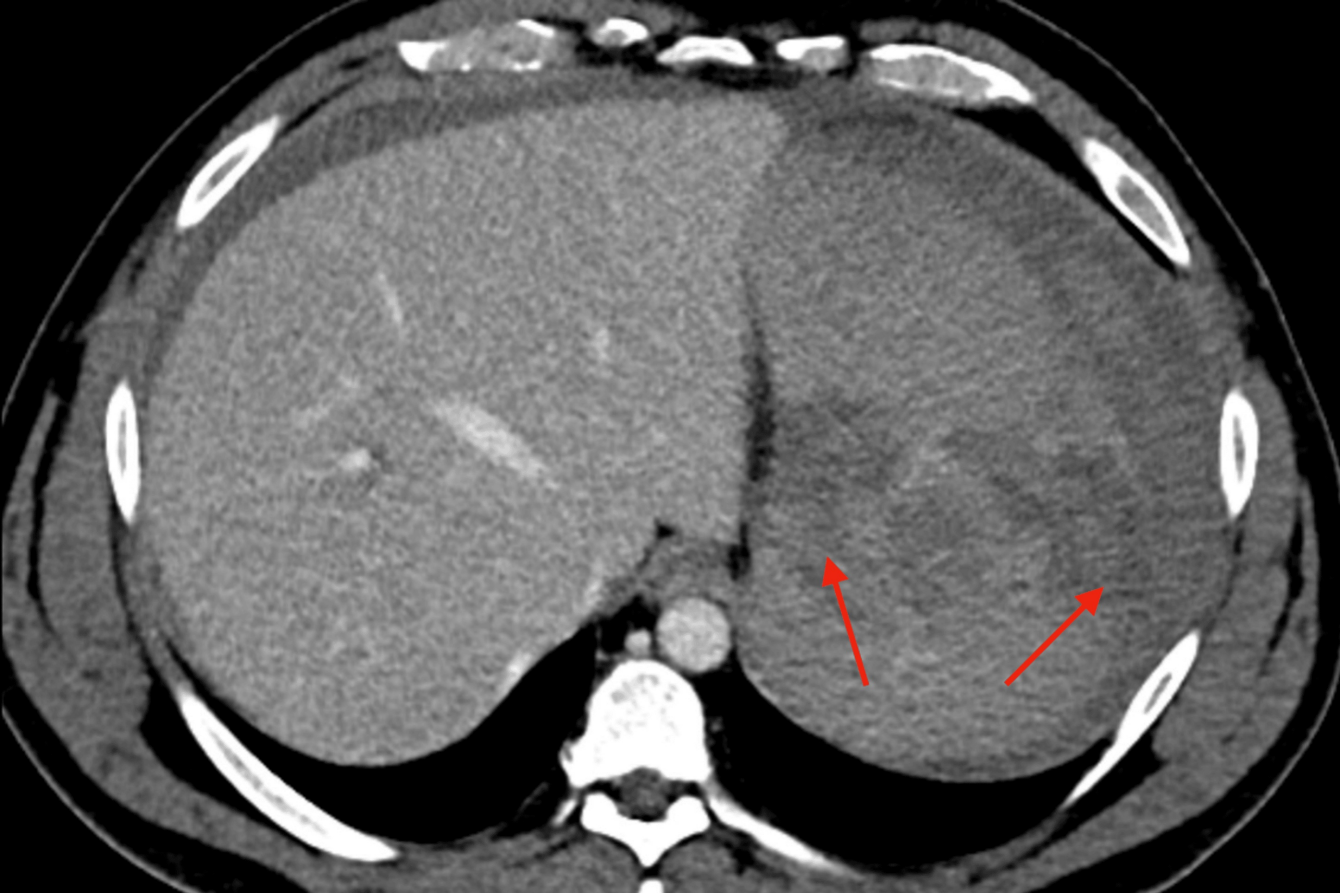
Axial contrast-enhanced CT findings of atraumatic splenic rupture Axial contrast-enhanced CT of the abdomen demonstrating irregular splenic contour with deep parenchymal lacerations (red arrows) and surrounding high-attenuation hemoperitoneum. CT: computed tomography
